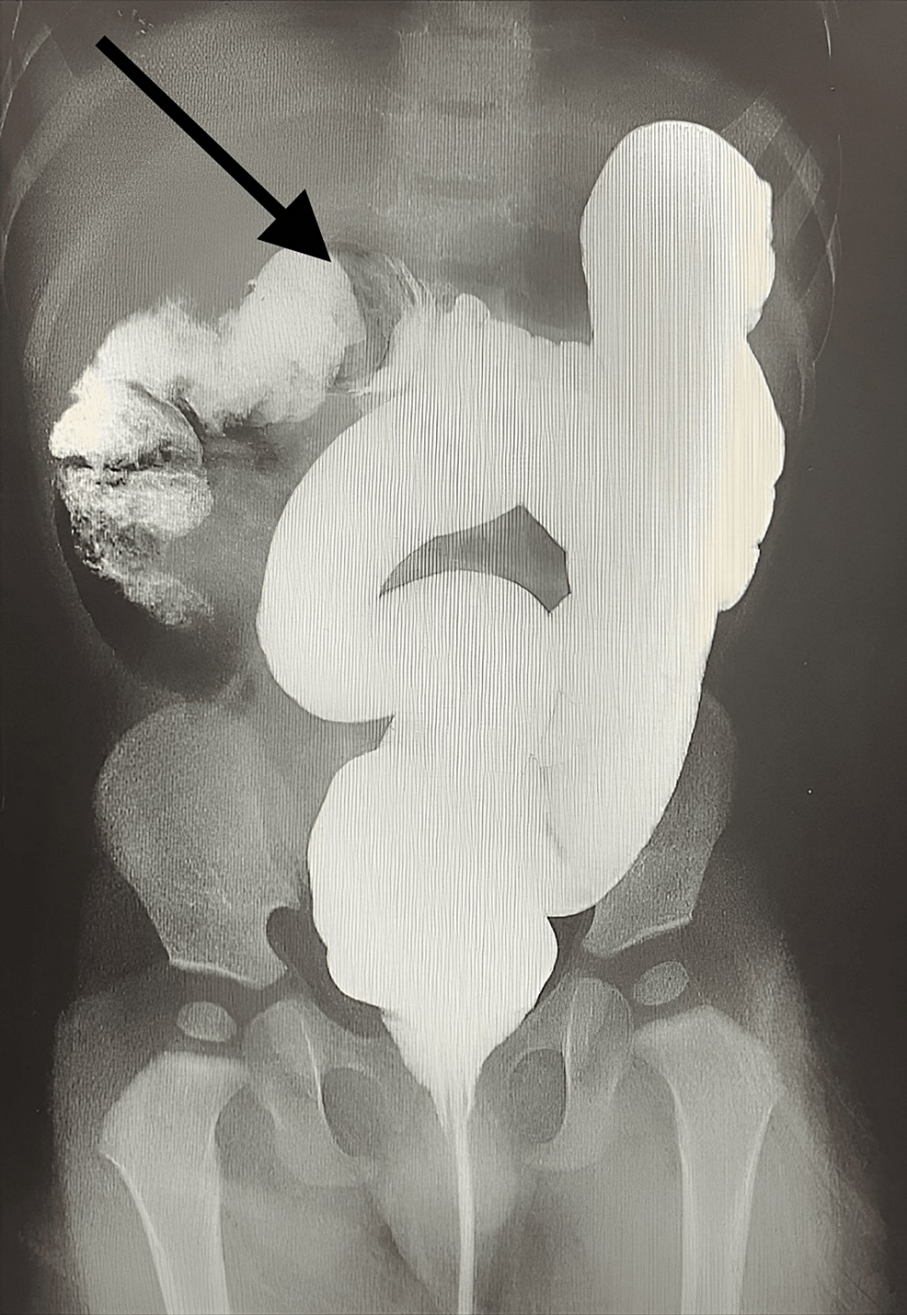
Day 2 X-ray gastrografin enema image showing the obstruction in the mid-transverse colon region
